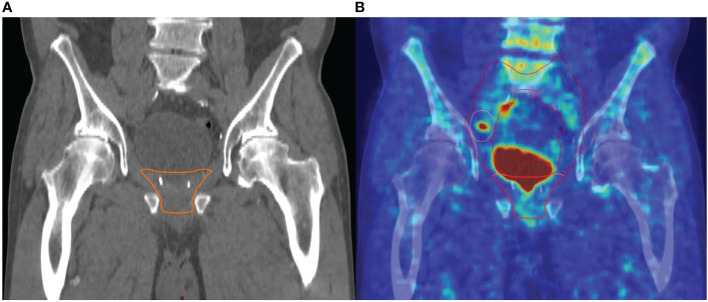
Example of major change of the radiotherapeutic treatment concept: (A) target volume without pelvic lymph nodes based on the clinical and pathological situation without PET/CT, (B) target volume with consideration of the PET/CT: additional irradiation of pelvic lymph nodes based on a PET/CT-positive right iliac lymph node metastasis.
